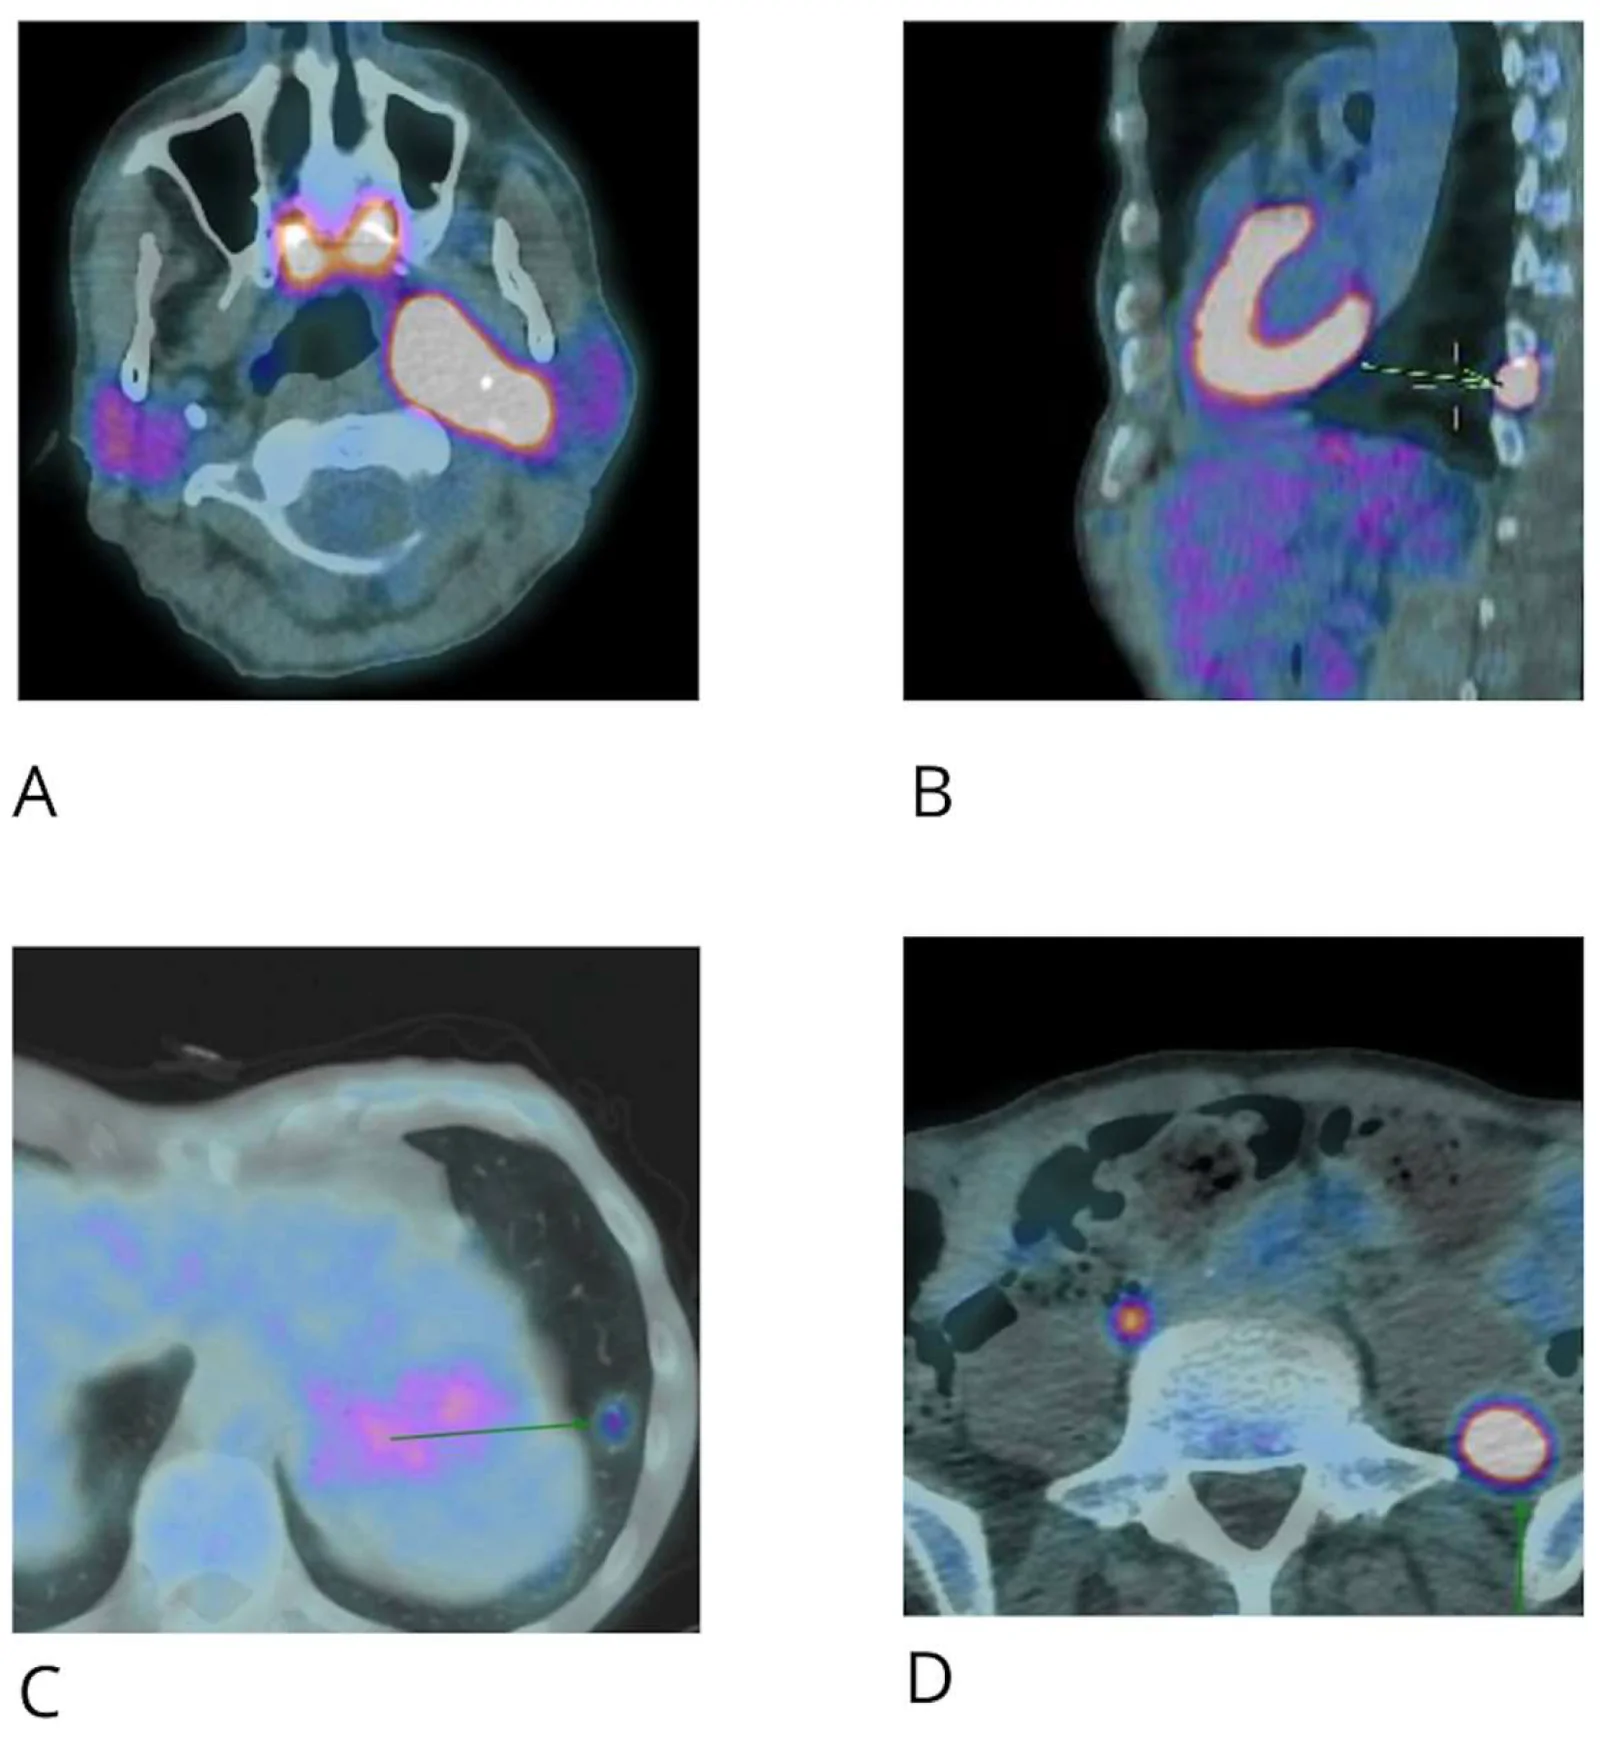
Postoperative staging FDG-PET. (A) Solid expansive lesion in the left submandibular region, showing continuity with the soft palate and extending cranially into the paranasopharyngeal space and caudally into the parapharyngeal and left level IIA cervical region (SUVmax 14.7, maximum diameter 5 cm). (B) Left paravertebral lesion at the level of the D11 vertebral body (SUVmax 8). (C) Pulmonary lesion in the basal lateral segment of the left lower lobe (SUVmax 4, maximum diameter 7 mm). (D) Focal nodal uptake in the left paramedian pelvic region (SUVmax 18.3, maximum diameter 3.5 cm) and additional uptake in the right iliac fossa (SUVmax 14).
